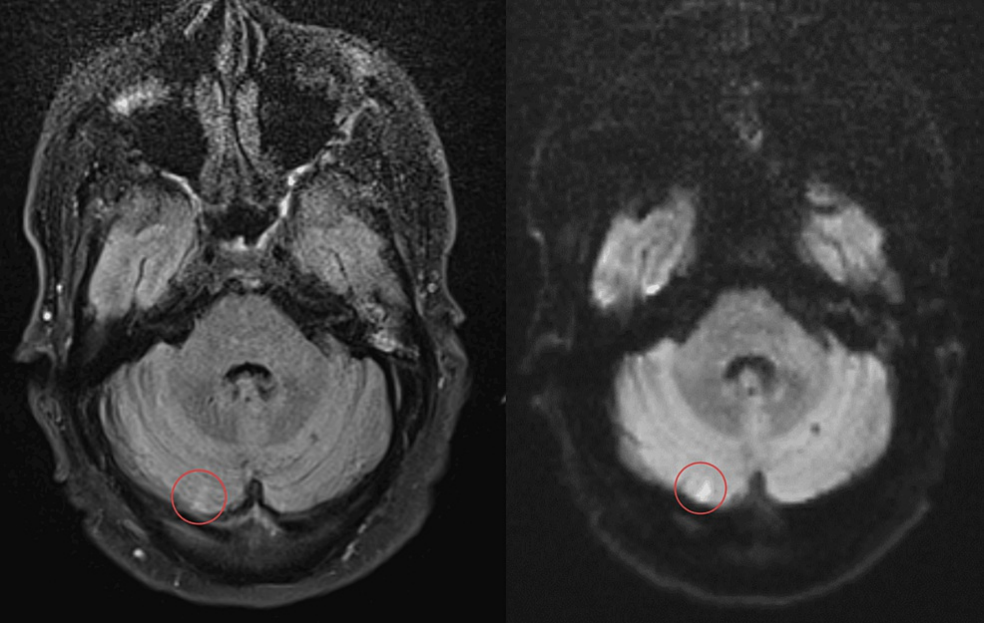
Brain MRI. Brain MRI showing a small focus of diffusion restriction within the right cerebellar lobe that likely represents a focus of acute/early subacute infarct, with no significant mass effect. MRI, magnetic resonance imaging
